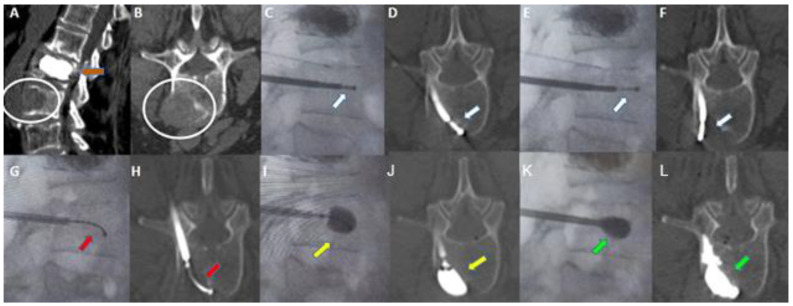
Sixty-eight-year-old woman with a history of breast cancer. (A,B): Sagittal (A) and axial (B) contrast-enhanced CT scan showing a metastatic lytic lesion of the left anterolateral portion of the L3 vertebra extending to adjacent perivertebral soft tissues (white circle in (A,B)). Previous vertebral augmentation treatment with Arcadia in L2 (orange arrow in (A)). (C,D): Fluoroscopy in the lateral plane (C) and axial CT scan (D). Placement of the STAR™ radiofrequency probe (white arrow) in the central portion of the lesion. (E,F): Fluoroscopy in the lateral plane (E) and axial CT scan (F). Placement of the STAR™ Radiofrequency steerable probe (white arrow) in the lateral aspect of the lesion. (G,H): Fluoroscopy in the lateral plane (G) and axial CT (H). Arcadia™ low compliance high pressure steerable balloon was inserted coaxially into the introducer cannula and deployed into the lesion (red arrow). (I,J): Fluoroscopy in the lateral plane (I) and axial CT (J). Arcadia™ low compliance high pressure steerable balloon gently inflated with a mixture of saline and iodinated contrast, with the intent of compacting the ablated tissue and creating a void for cement delivery (yellow arrow). (K,L): Fluoroscopy in the lateral plane (K) and axial CT (L). StabiliT^®^ high viscosity bone cement was slowly delivered into the lesion for the stabilization of the vertebral body (green arrow).
